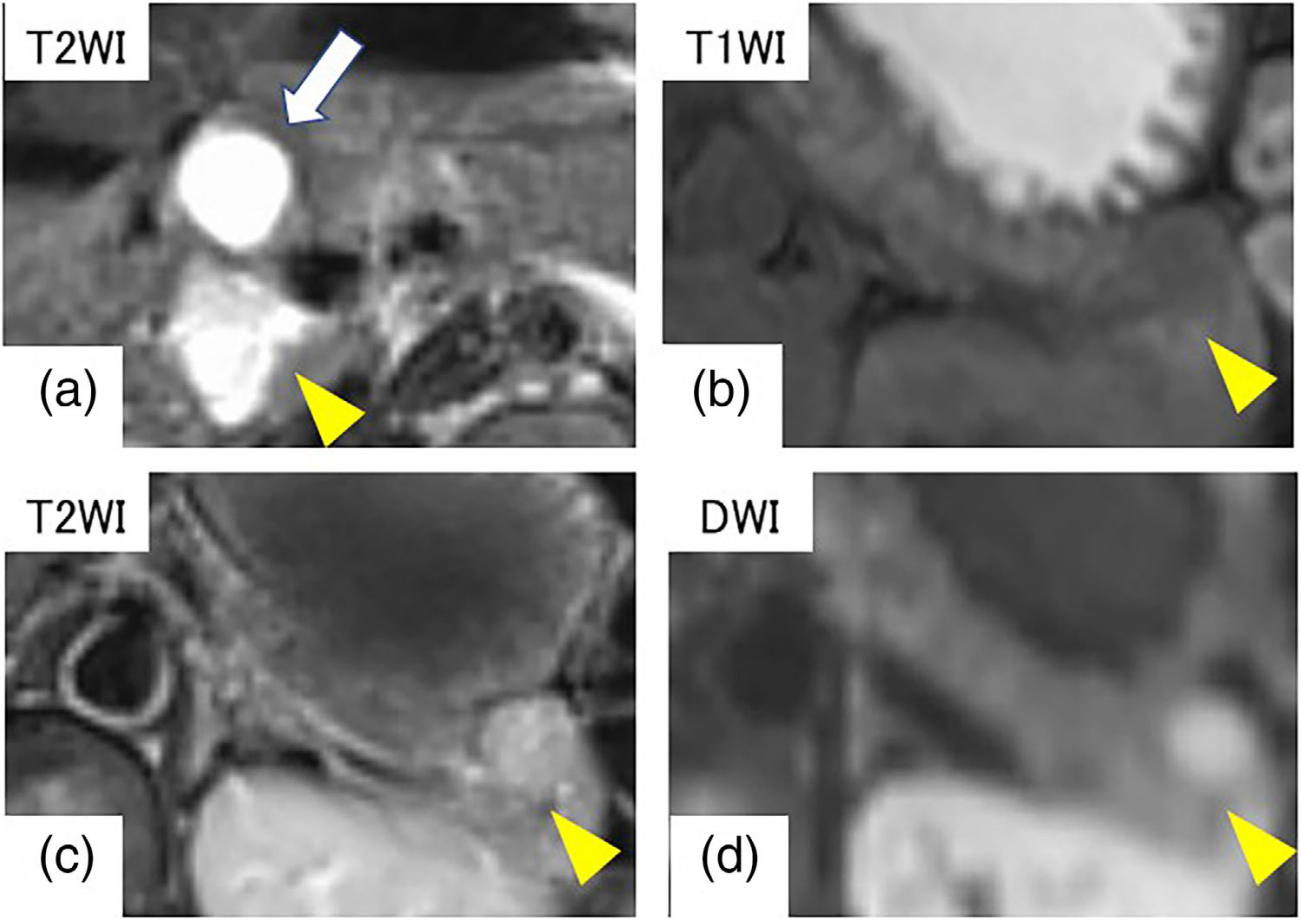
Magnetic resonance imaging. (a) The lesions in the pancreatic head (yellow arrowhead) and body (arrow) were very hyperintense in T2‐weighted imaging. (b–d) The lesion in the pancreatic tail was hypointense in T1‐weighted imaging, hyperintense in T2‐weighted imaging, and hyperintense in diffusion‐weighted imaging.
